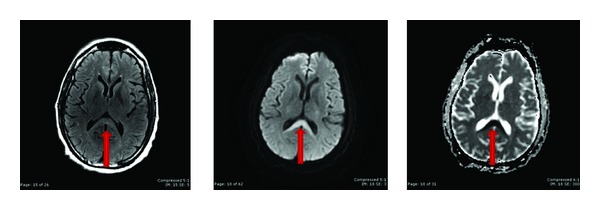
FLAIR and DWI sequences with corresponding ADC map at the level of corpus callosum splenium show hyperintense T2 signal and diffusion restriction compatible with cytotoxic edema.
